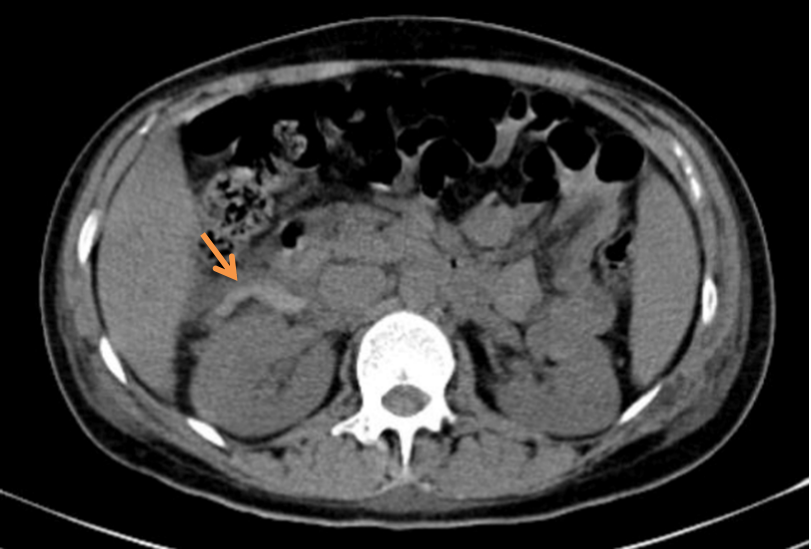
Computed tomography scan showed a tortuous right ovarian vein with surrounding amount of fluid suggestive of ovarian vein thrombosis.
